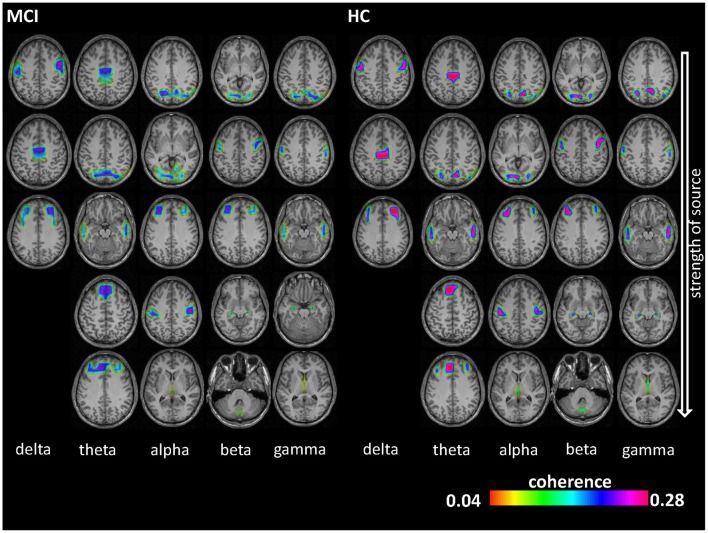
Electroencephalography (EEG) coherence sources results. Coherence sources are shown for the five examined frequency bands: delta (1–3 Hz), theta (4–7 Hz), alpha (8–13 Hz), beta (14–30 Hz) and gamma (30–49 Hz). Coherence can range from 0 to 1 (i.e., two sources have temporally fully aligned time courses). Sources are displayed on a template T1-weighted brain. The first source (1) in each frequency band is the highest power source and was later used as reference for determining the subsequent coherent sources.
